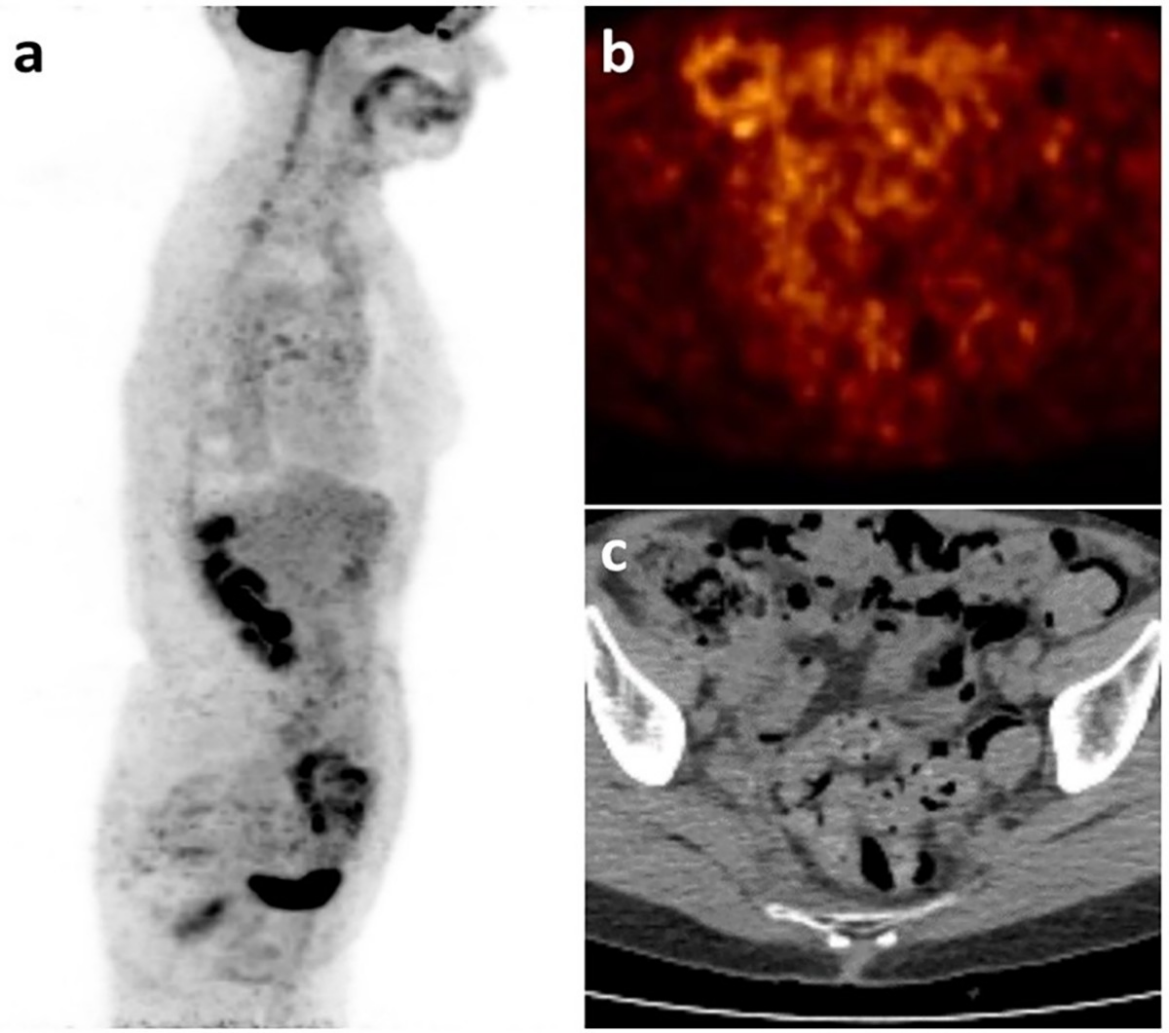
Peritoneal carcinosis undetected at PET/CT. Patient with locally advanced ductal breast carcinoma (pT2, N2a, M0) after surgery and 4 cycles of chemotherapy, under endocrine therapy. After suspicious rise in CA15-3 the patient underwent FDG-PET/CT, which showed no suspicious uptake and was reported as negative for distant metastases. Sagittal MIP (a) showed non-specific FDG uptake in the ascending colon and tracer excretion in the urinary tract. Pelvic axial cross-section of the FDG-PET/CT (b) and the co-registered CT image (c) did not show any suspicious uptake or measurable lesion.
